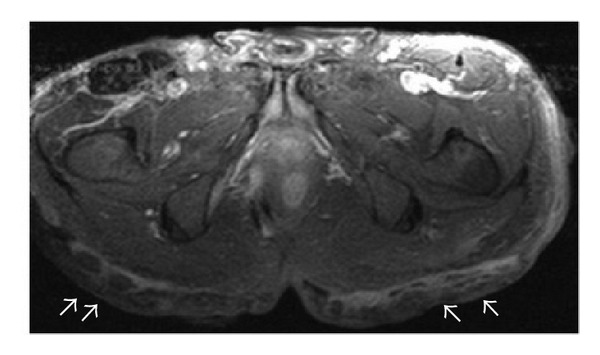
Axial T1-weighted fat-suppressed image postintravenous Magnevist: this MRI image done at the patient's first presentation 9 years ago shows multiple rim-enhancing subcutaneous abscesses in bilateral gluteal regions (white arrows) without deep extension or significant dermal induration, consistent with the early course of the disease.
